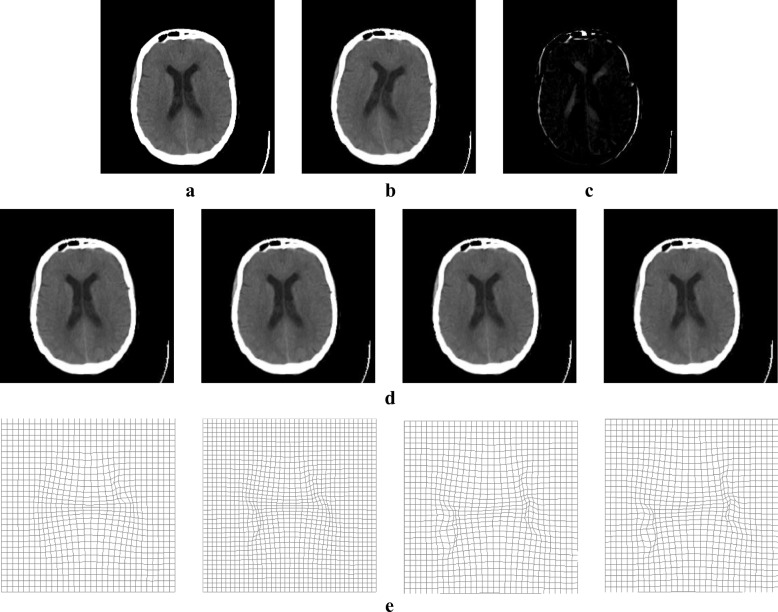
Registration result of CT image: (a) reference image; (b) moving image; (c) initial difference between reference and moving images; (d) registration results obtained using our method, diffeomorphic demons, additive demons, and active demons, respectively, from left to right; and (e) final deformation field
